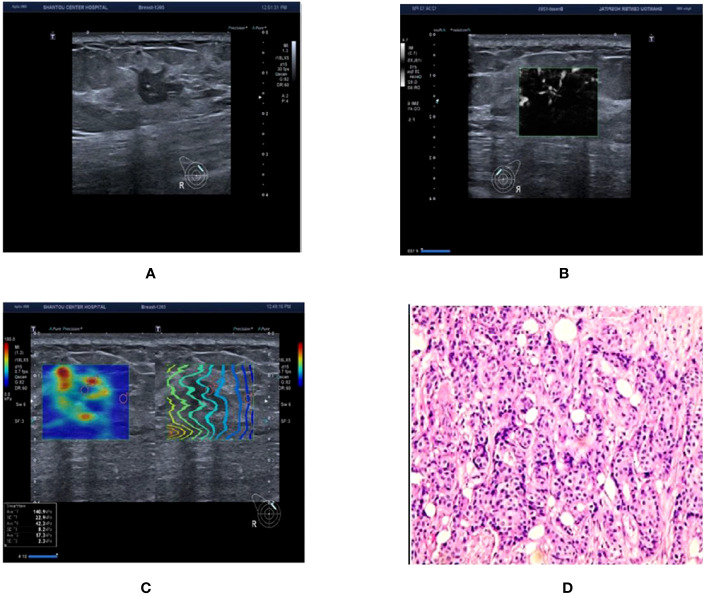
Ultrasound and pathological graphs: A 50-year-old female patient with a right breast nodule received ultrasound and pathological biopsy examinations. (A) An irregularly shaped, crab-like hypoechoic nodule is detected in the upper outer quadrant of the right breast, with unclear boundaries, classified as BI-RADS 4c. (B) mSMI image demonstrates that the microvascular morphology is radicle-shaped, with an increased BI-RADS level of 1 classified as BI-RADS 5. (C) Shear wave elastography image: Emax is 140.9 kPa, which is higher than 77.25 kPa, resulting in an increase or no change in the BI-RADS classification. (D) The nodule is an invasive ductal carcinoma based on pathological results.
